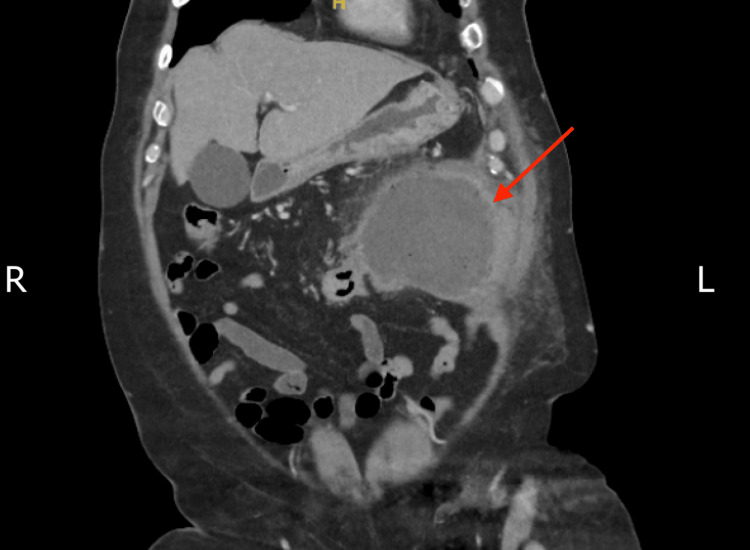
Coronal contrast-enhanced CT scan showing an infected collection in the left anterior abdominal wall (red arrow).
